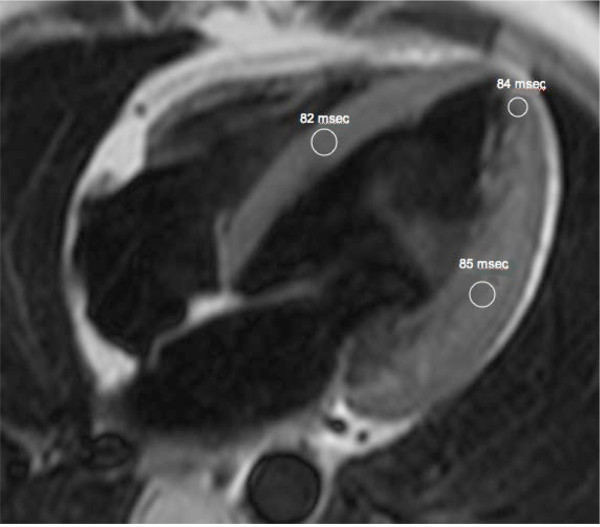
Four-chamber horizontal long-axis T2-weighted black blood turbo spin-echo MR image. White circles outline regions of interest in the mid septum, apex, and infero-lateral wall. MT2RTs were 82 milliseconds in mid septum, 84 milliseconds in apex, and 85 milliseconds in infero-lateral wall.
